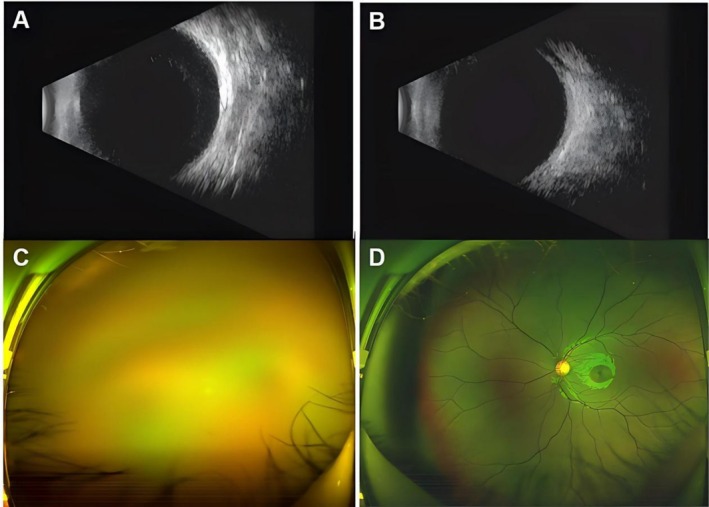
B‐scan ultrasonography and fundus photography findings. (A, B) B‐scan of the right eye revealed moderate vitreous opacity and the left eye showed mild vitreous opacity. (C) Fundus photography of the right eye was obscured. (D) Fundus photography of the left eye showed no obvious abnormalities.
